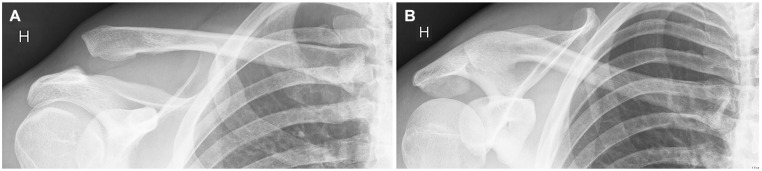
(A) Nonweightbearing anteroposterior (AP) radiograph of the acromioclavicular (AC) joint; a 15° cephalic tilt in the vertical plane. (B) Nonweightbearing AP radiograph of the AC joint in the same patient; a 15° caudal tilt in the vertical plane.
